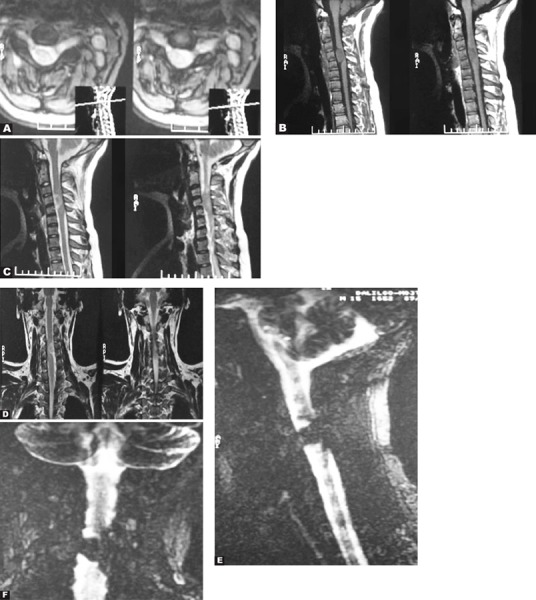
A 25-year-old man with weakness and paresthesia in his upper limbs. There is also an intra-dural extra-medullary mass with compressive effect on the adjacent cord. A. Axial T2-weighted MR image B. Sagittal T1-weighted without contrast C. Sagittal T2-weighted image D. Coronal T2-weighted image. E. MR Myelogram, sagittal view. F. MR Myelogram, coronal view.
